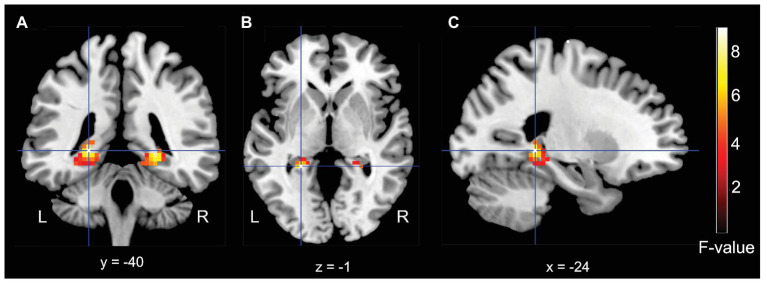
Results for the medial-temporal volume of interest analysis. (A) Coronal, (B) Axial, and (C) Sagittal (left) MRI brain slices depicting a bilateral posterior hippocampal regions that showed a significant interaction effect for the factors of Familiarization (non-familiarized vs. familiarized) and Arrangement (single object vs. pair).
